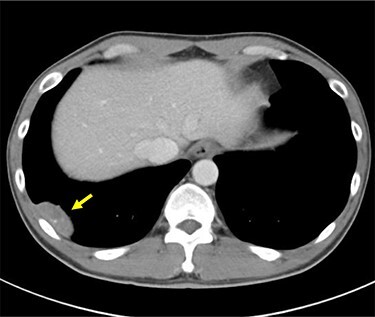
Preoperative enhanced CT scan demonstrates a well-defined 44 mm solid nodule with calcification in the right lateral thoracic cavity (yellow arrow). CT: computed tomography.
